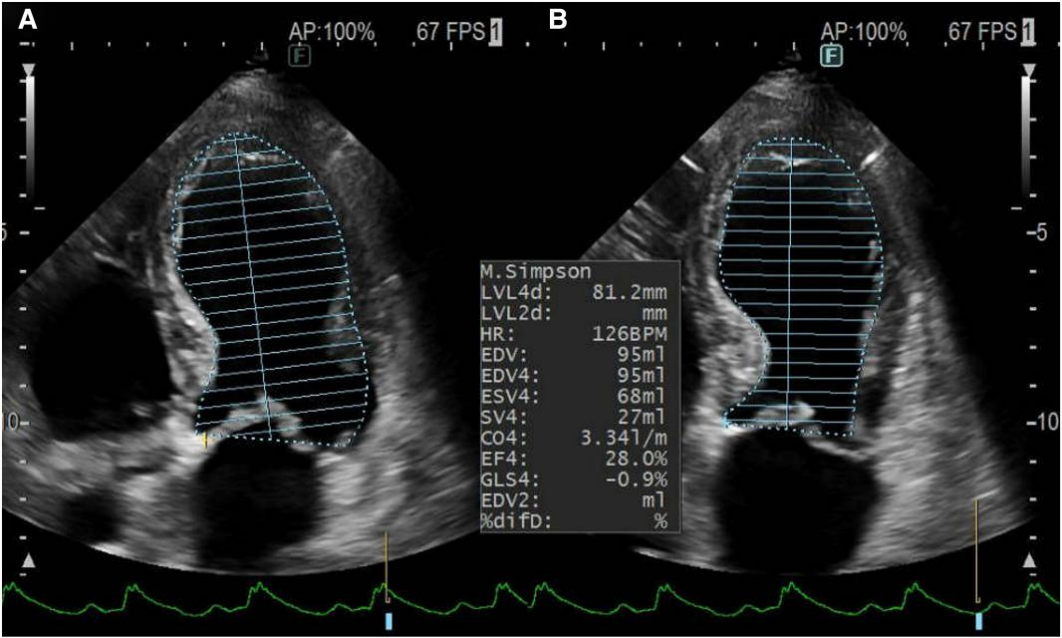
Transthoracic echocardiography demonstrating severely reduced left ventricular ejection fraction (28%, Simpson) with mid-to-apical hypokinesia and apical ballooning. (*A*) Diastolic phase and (*B*) systolic phase.
